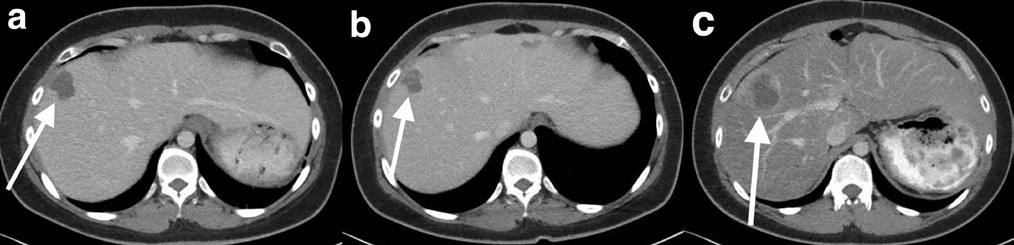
Recurrent liver metastases. Portal venous CT image demonstrates a treated segment 8 liver metastasis with expected cystic attenuation (a). CT performed 3 months later shows a similar sized lesion with new areas of internal enhancement anteriorly and centrally (b). A third CT performed 6 months later demonstrates marked increased density and size increase in size in keeping with recurrent metastatic disease related to second-line tyrosine kinase inhibitor resistance (c).
